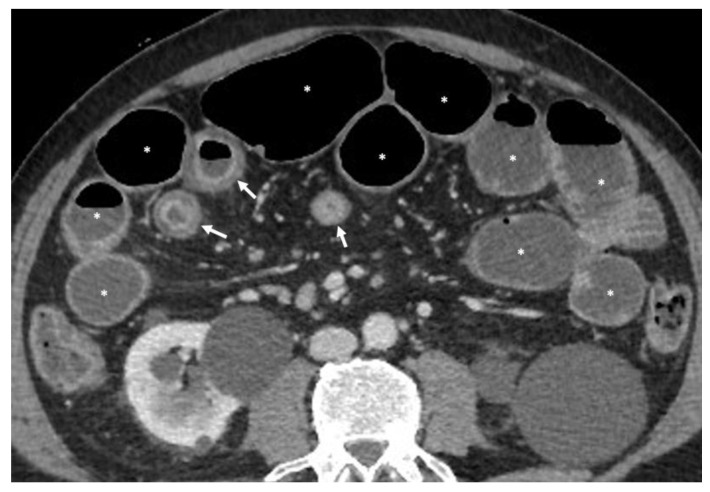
Axial CT image after injection of contrast medium, performed at the emergency department, shows wall thickening of some small bowel loops in right abdomen (arrows), due to Crohn’s disease, which is causing upstream bowel dilatation (asterisks).
